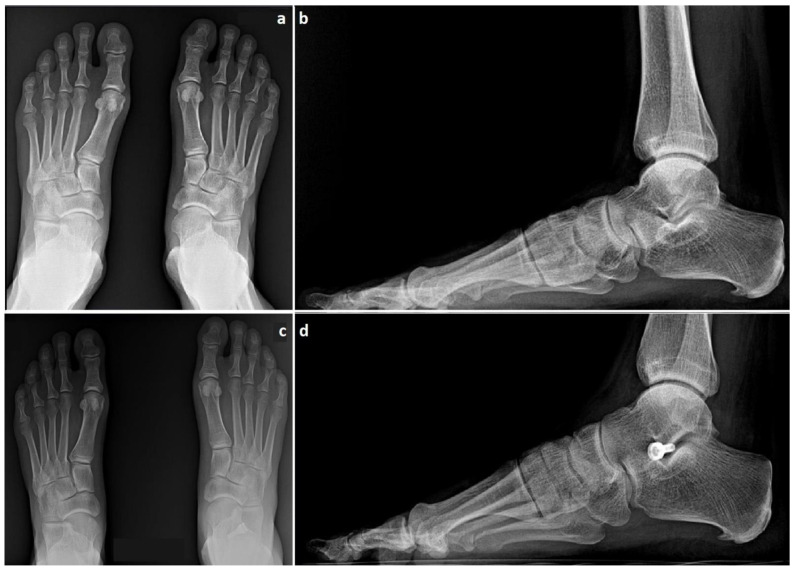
Foot radiographs in a–p (anterior–posterior) and lateral projections before surgery (a,b) and during the follow-up visit 12 months after surgery (c,d). All imaging examinations were performed in a standing position.
